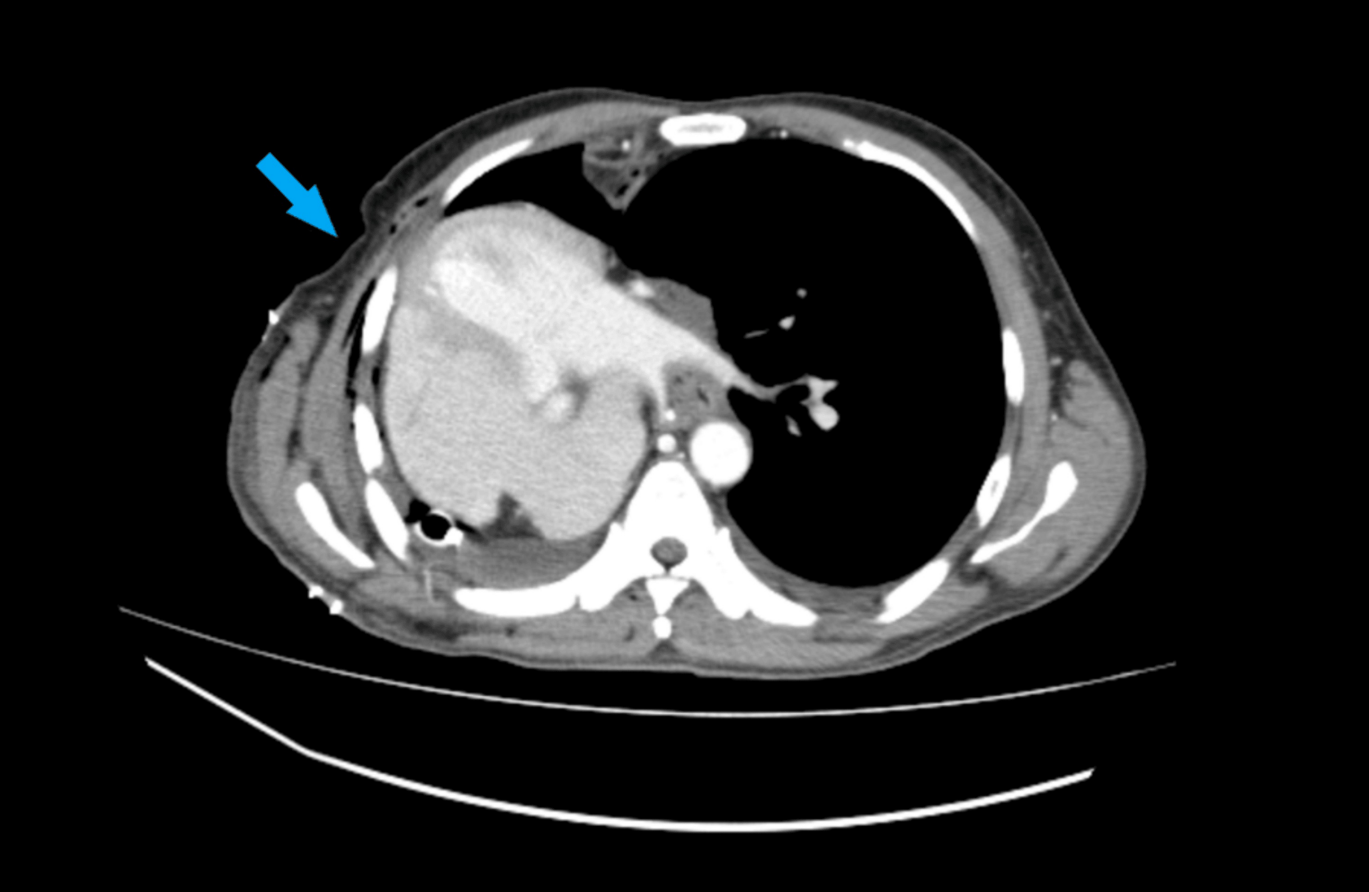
Axial contrast-enhanced CT image of the thorax Axial contrast-enhanced CT image of the thorax at the level of the left ventricle demonstrating complete right mediastinal shift (blue arrow), with the left ventricular apex facing laterally. The right atrium and right ventricle are located posteriorly, suggesting up to 180-degree axial cardiac rotation on its axis. Small bilateral pleural effusions, right chest tube, and pulmonary veins are also visualized. CT: computed tomography
